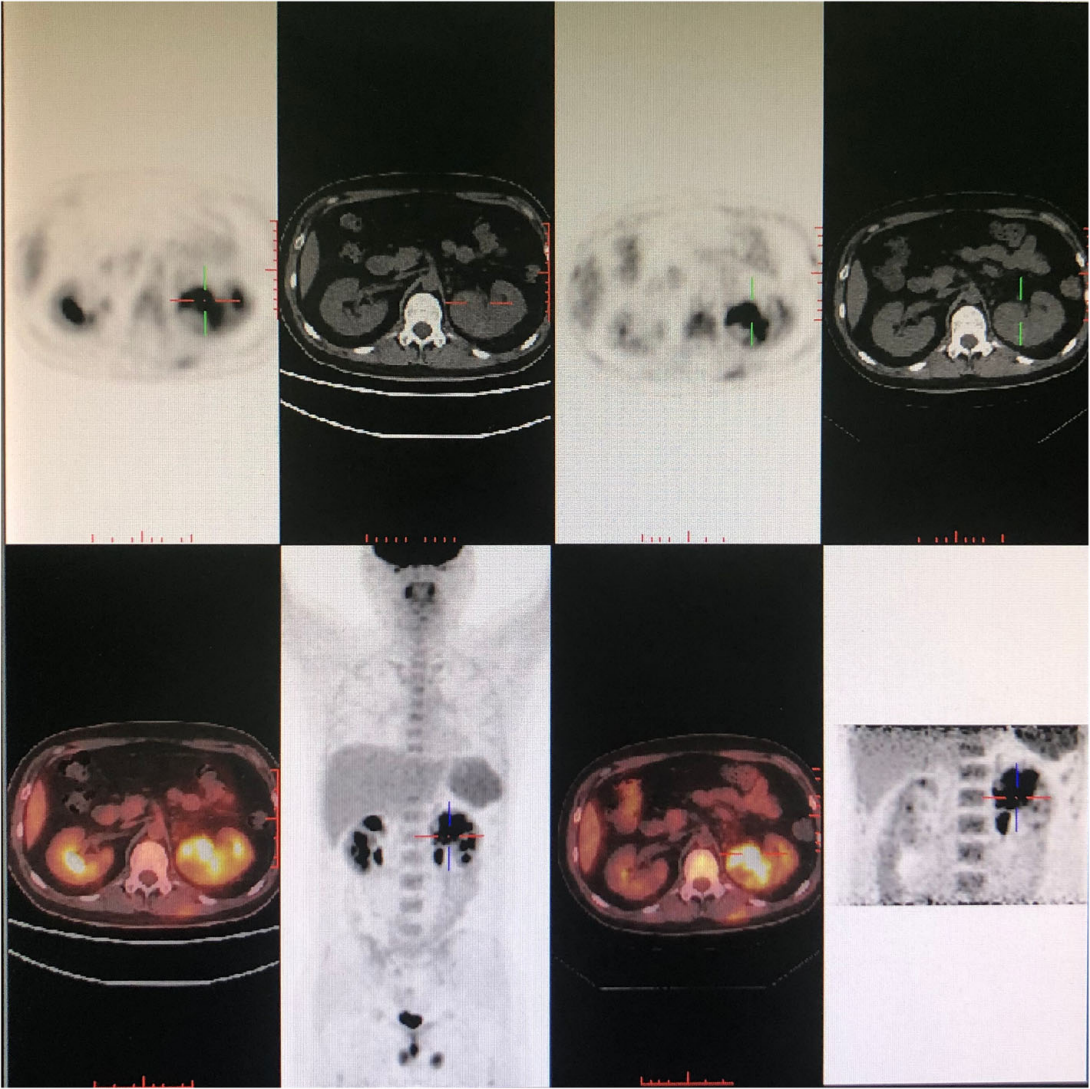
PET/CT scan on reveals an occupying lesion, approximately 4.7 × 5.7 × 5.1 cm in size, in the upper pole of the left kidney. The early SUVmax is 14.5 and the delayed SUVmax is 21.7. The upper segment of the left ureter is thickened and occluded
